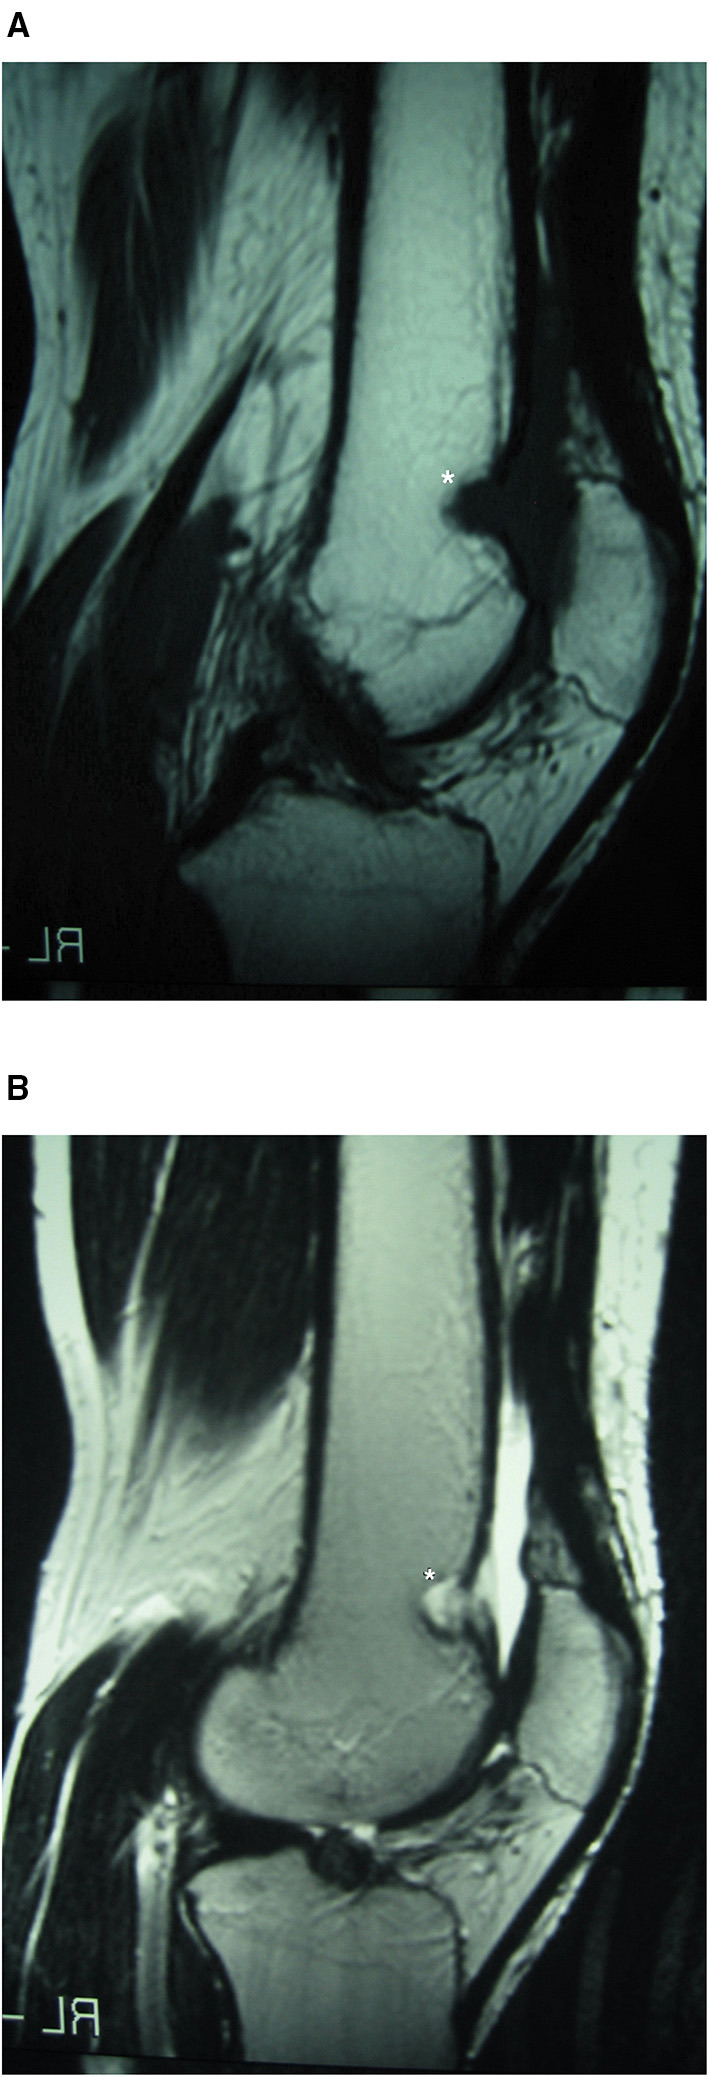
Case 1: suprapatellar SH MRI. Conventional sagittal T1 (A) and T2 (B) MRI showing synovial haemangioma in the suprapatellar and supratrochlear synovial lining extending medially and eroding the supratrochlear cortical bone, with features of hyperintensity on T2-weighted imaging and hypo-/isointensity to muscles on T1. *Location of the Synovial Haemangioma tumor in the knee.
